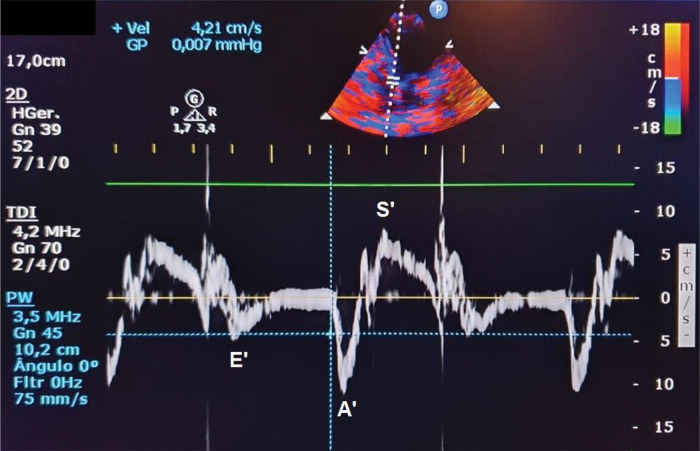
Septal tissue Doppler in the apical window four-chamber view to assess the velocity of mitral annular systolic (S’) and diastolic excursion in the early filling phase (E’) and atrial contraction phase (A’).
